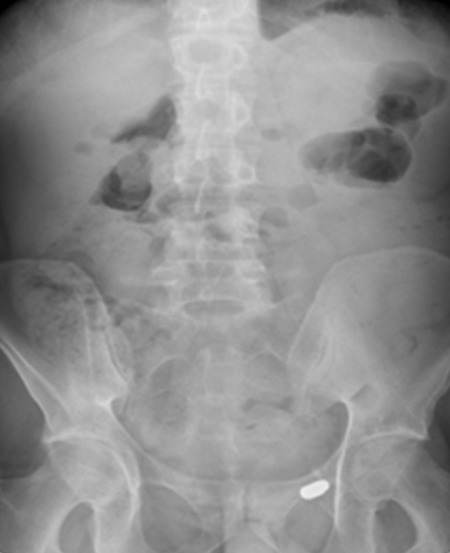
Abdominal radiography with pneumoperitoneum
